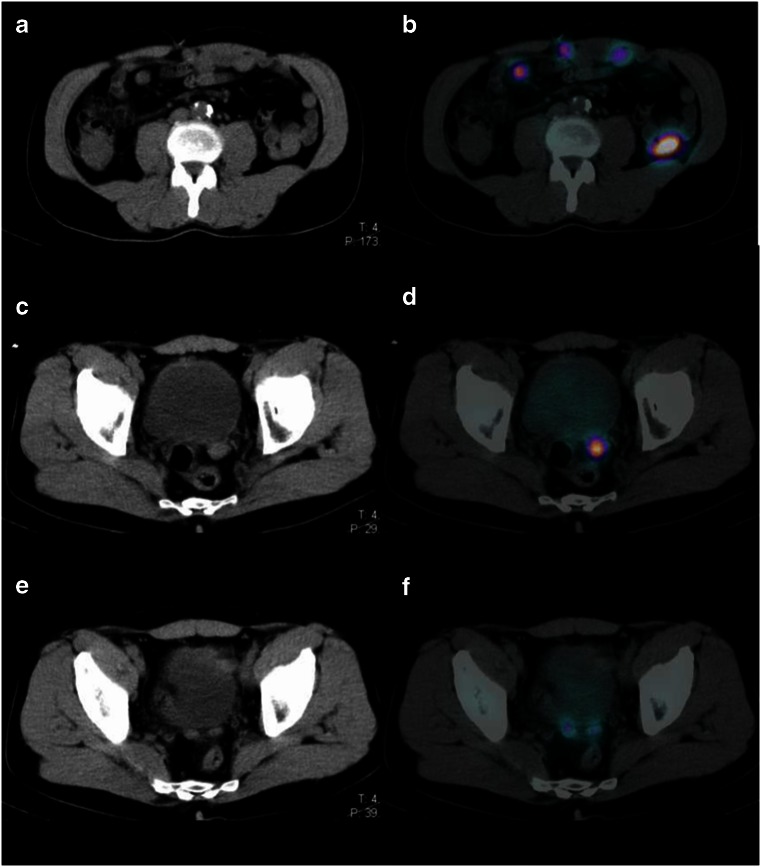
a–f CT of the abdomen and pelvis with Tc99m colloid SPECT overlay, demonstrating avid uptake of tracer
